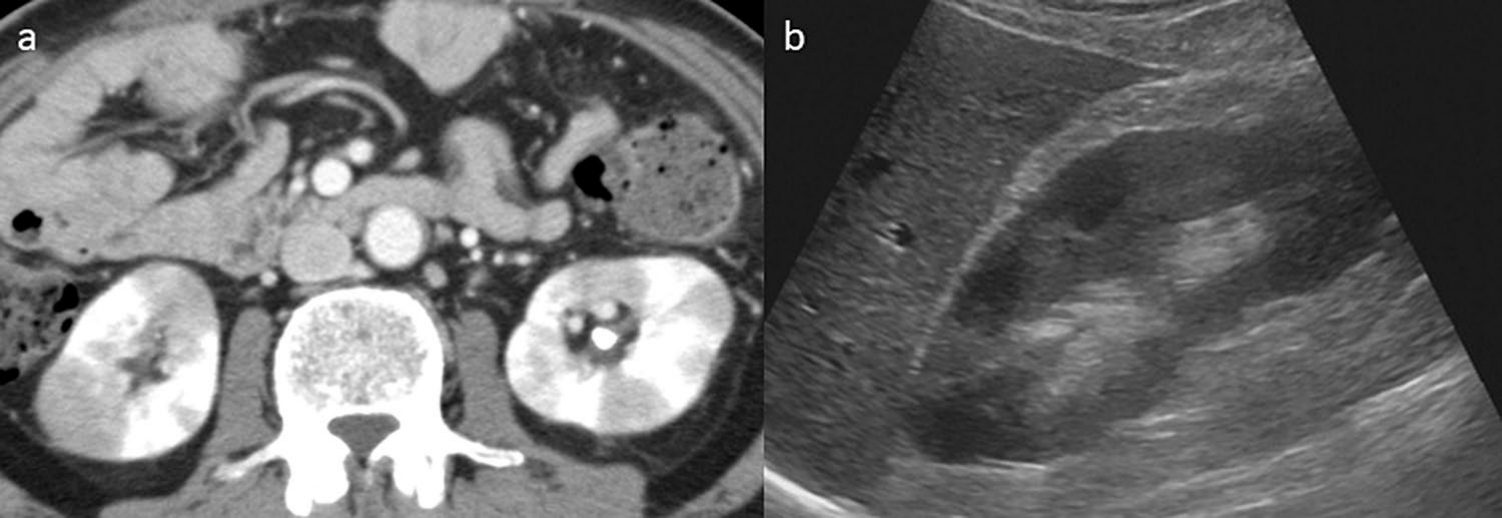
Comparison of the radiological findings of renal lesions between contrast-enhanced CT and transabdominal ultrasound in a patient with AIP. (a) A contrast-enhanced CT showing multiple hypodense lesions in both kidneys. (b) Transabdominal ultrasound showing multiple ill-defined, non-mass-like areas with decreased echogenicity in the right kidney.
